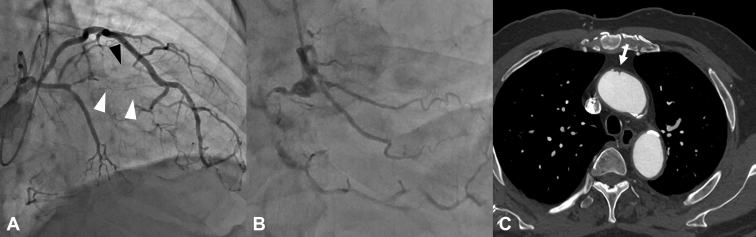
A, Coronary angiography demonstrated total occlusion of the left anterior descending artery (*white arrowhead*) and the diagonal branch (*black arrowhead*). B, Severe stenosis was observed at the ostium of the right coronary artery. C, Computed tomography demonstrated atheromatous changes in the ascending aorta (*white arrow*).
